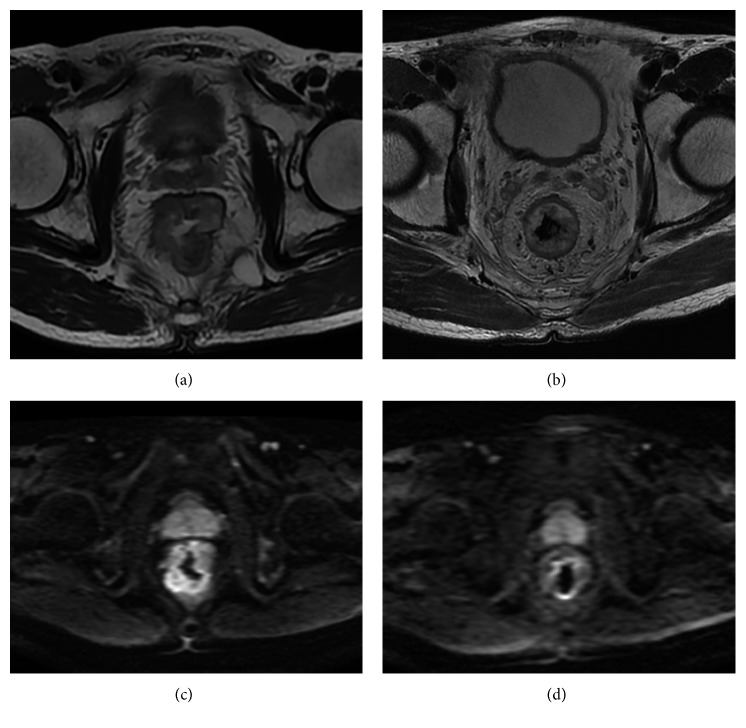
A 68-year-old man with bloody stool for more than 2 months. (a, b) Baseline conventional MRI before treatment. (c, d) Conventional MR images after treatment. (a) HR-T2WI transverse axial image shows marked uneven thickening of the rectal wall. (b) Axial DWI image shows increased DWI signal of the thickened intestinal wall, with an ADC value of 0.82 × 10^−3^ mm^2^/s. (c) HR-T2WI transverse axial image shows edema of the rectal wall and increased signal intensity. (d) Axial DWI image shows that the DWI signal of the intestinal wall is significantly lower than before, with an ADC value of 1.11 × 10^−3^ mm^2^/s.
